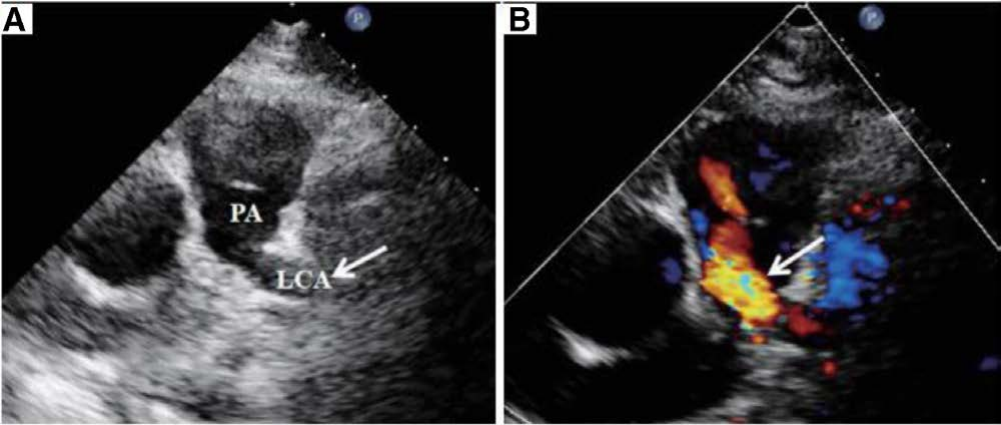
(A) Echocardiography shows the LCA originating from the posterior wall of the pulmonary trunk (arrow); (B) The blood of the LCA flows back into the PA.^[7]^ LCA = left coronary artery, PA = pulmonary artery.
